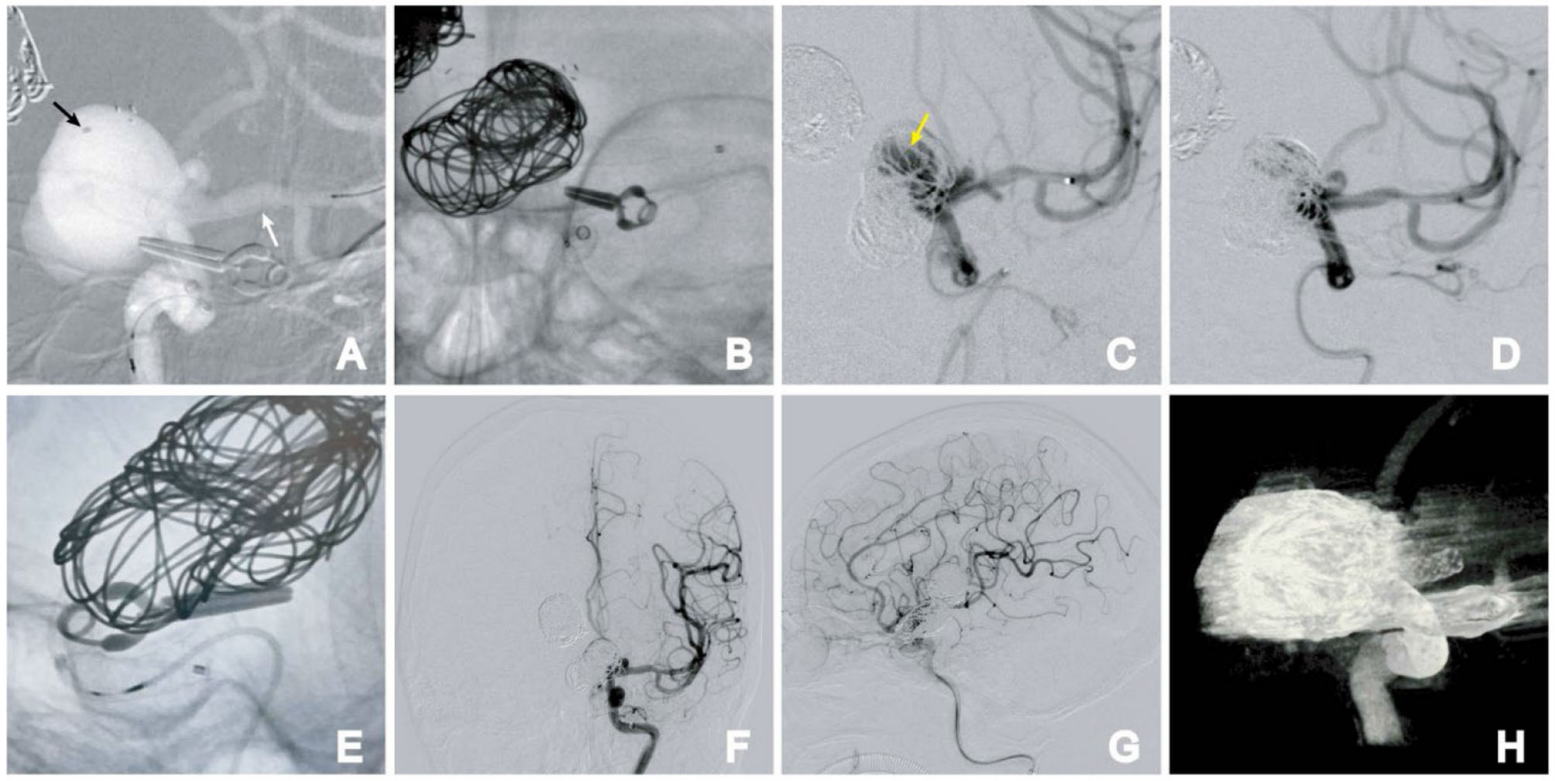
Stage 2. (A) Semi-deploying PED; PED anchor point (white arrow); coil catheter position (black arrow); (B) large-sized coil embolization was performed, followed by the complete deployment of the PED; (C) angiographic images reveal direct blood flow (arrow); (D) direct blood flow disappeared after further embolization of the aneurysm; (E) PED was fully deployed. (F, G) Postoperative frontal (F) and lateral (G) DSA images. (H) Dyna-CT showing the PED was fully deployed.
